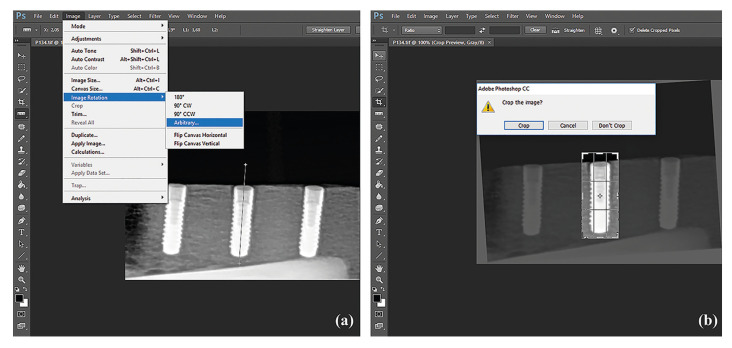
Processing the panoramic radiographs to be evaluated. (a) The long axis of the implant was defined, and the image was rotated. (b) A rectangular bounding box was drawn, and images were cropped separately.
